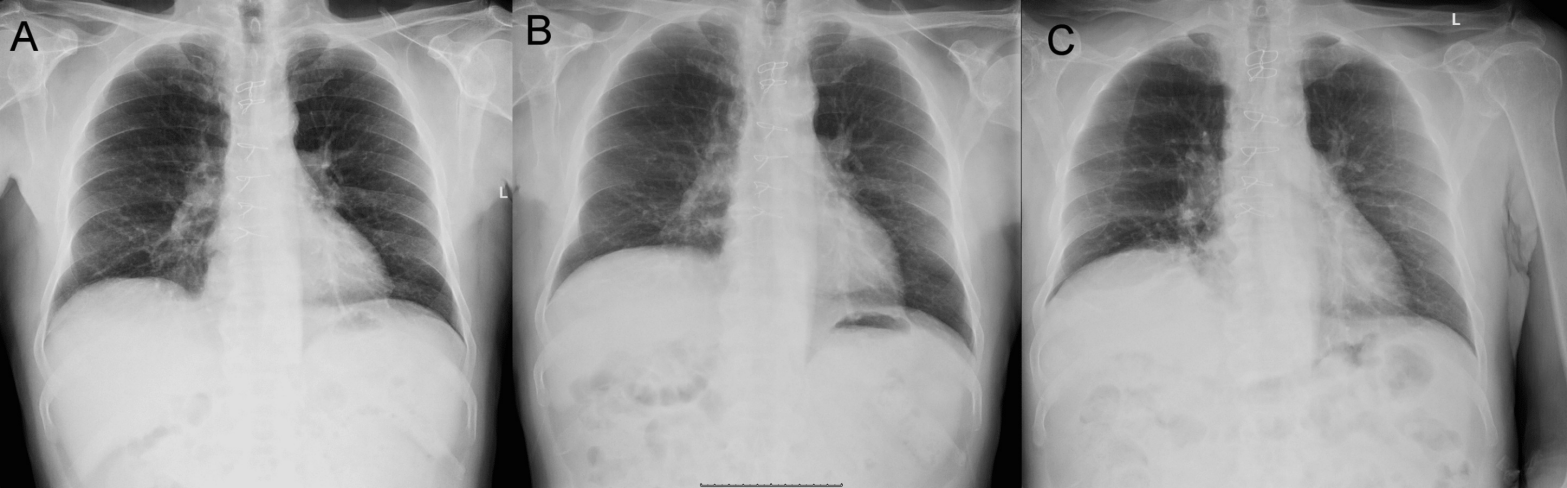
CXR time series after CABG: (A) 2007, (B) 2012, and (C) 2021 The postoperative CXR in 2007 (A) showed no evidence of hemi-diaphragmatic elevation, while the CXR performed in 2012 (B) showed an intermediate position. The raised hemidiaphragm was still present in 2021 (C) CXR: chest X-ray; CABG: coronary artery bypass grafting
